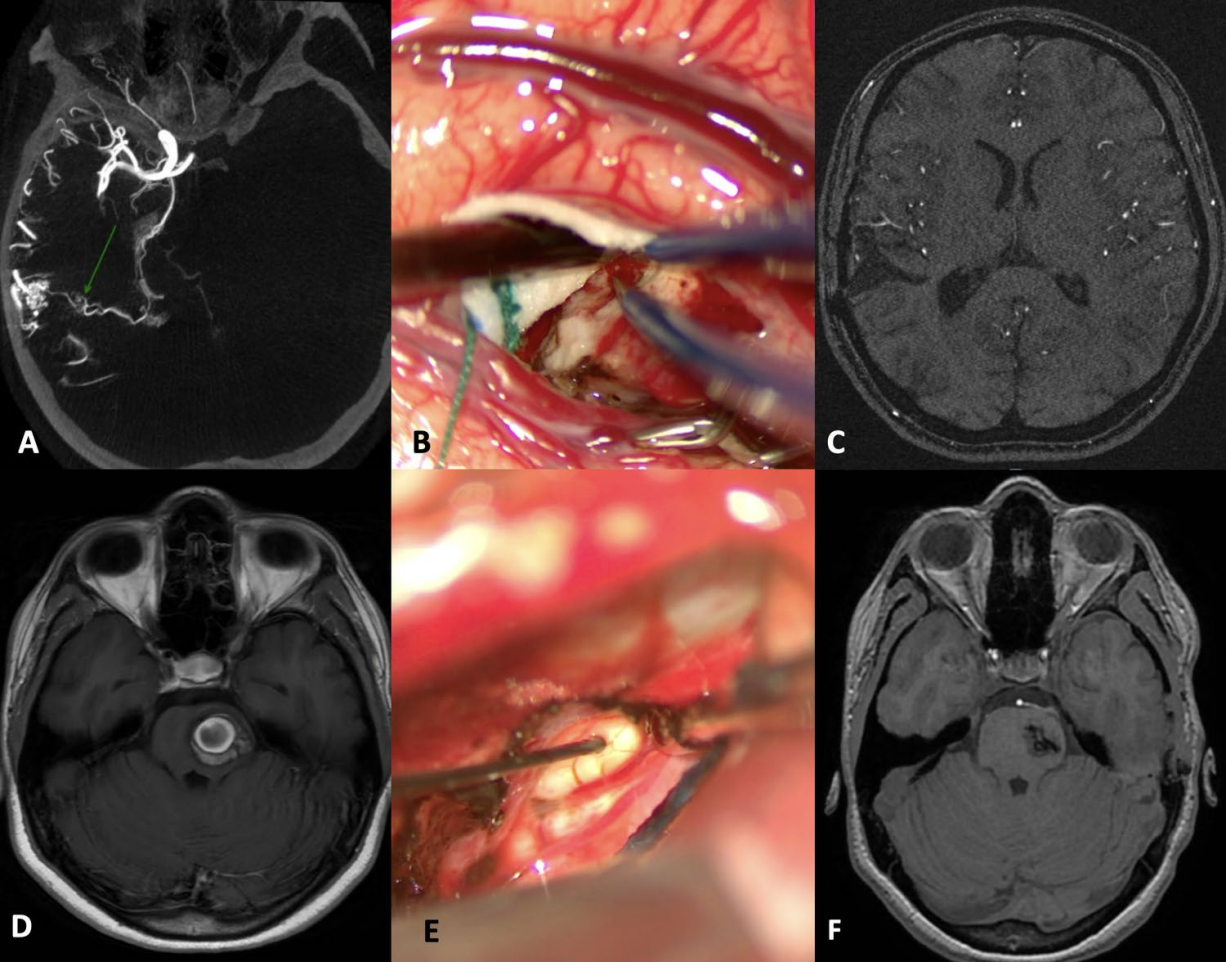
A A 16-year-old patient (*AVM case number 4*) presenting with intraparenchymal hematoma in the right temporal lobe sustained by a compact-nidus AVM fed by distal branches of the right MCA and draining into the ipsilateral Labbé vein. B The patient underwent surgery with MEP and SSEP monitoring and the use of a subcortical stimulator. The nidus was circumscribed with the aid of a stimulated aspirator (no response evoked with stimulation up to 12 mA) and by coagulating the comb-like branches until the feeder originating from the middle cerebral artery was identified at the base. C At the 2-year follow-up, complete excision of the malformation is confirmed and clinically the patient presents no neurological deficits. D A 17-year-old patient (*cavernoma case number 2*) was diagnosed with a cavernous malformation in the left pons after an episode of severe headache. An MRI at 3 months showed an increase in the size of the lesion with signs of recent bleeding. She therefore underwent surgery through a left subtemporal approach. E Mapping of the midbrain surface was performed to identify the entry zone without sensory-motor responses. The dissection was performed with simultaneous mapping and neurophysiological monitoring to ensure safe excision of the malformation. F A follow-up MRI scan at 6 months documented complete removal of the cavernoma and the patient showed an improvement of the hyposthenia presented after surgery, with independent walking possible
